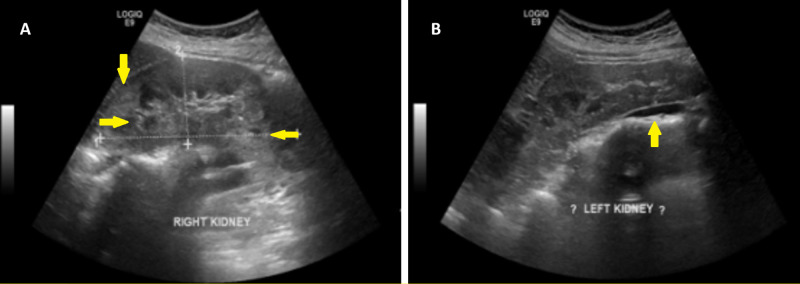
Ultrasound revealing multiple bilateral renal angiomyolipomas in the (A) right kidney and (B) left kidney
